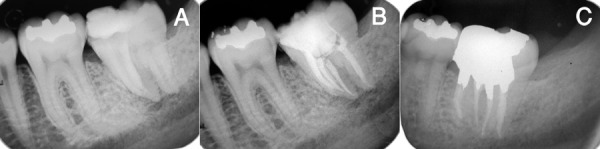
A) Periapical view of the left lower second molar, B) Final periapical radiography, C) Periapical view on one-year follow up
